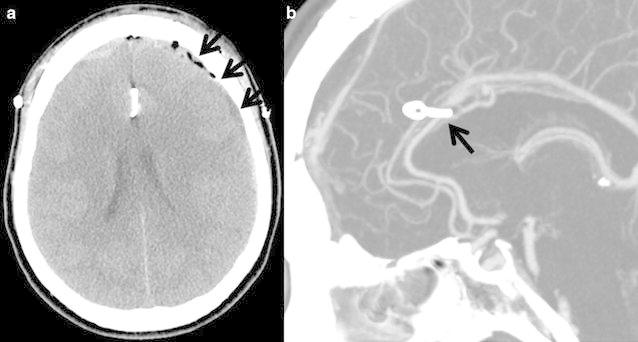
a, b Postoperative CTA revealed that previous subdural hematoma was evacuated and aneurysm was clipped without a remnant sac (*arrow*)
